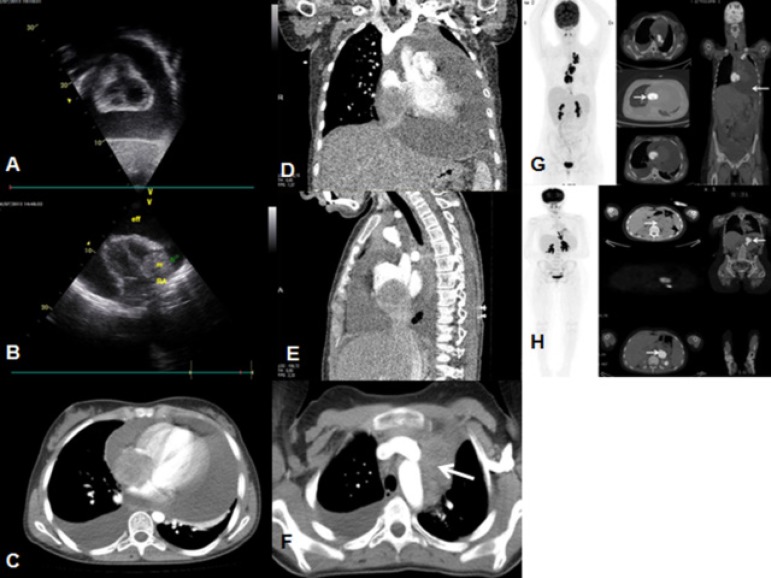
A) Pericardial effusion in transthoracic echocardiography. B)
